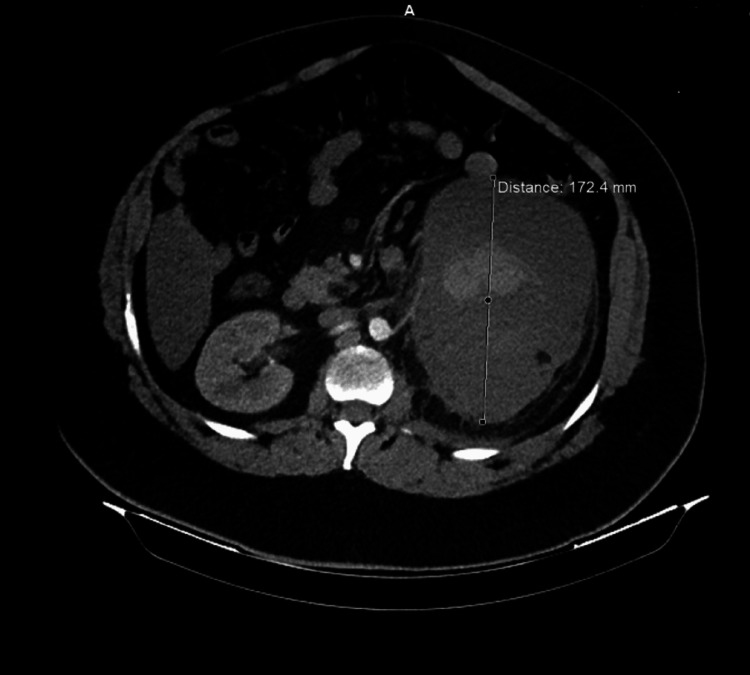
Hematoma with a diameter of 17 cm confined within the perirenal fascias on contrast-enhanced computed tomography scan.
